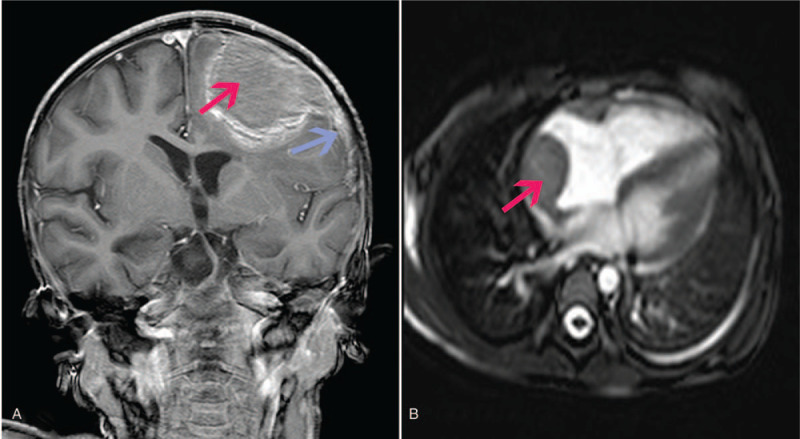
A. Brain MRI enhancement imaging showed mild enhancement with a wide base connected to the meninges (blue arrow). The MRI showed a round-like mixed signal shadow on the left frontal and parietal part on the sagittal position, mainly presented with T2 equal signal, and the surrounding parenchyma was slightly compressed (red arrow). B. MRI of heart also showed an isodense on T1WI or T2WI on the top of the right atrium. MRI = magnetic resonance imaging.
